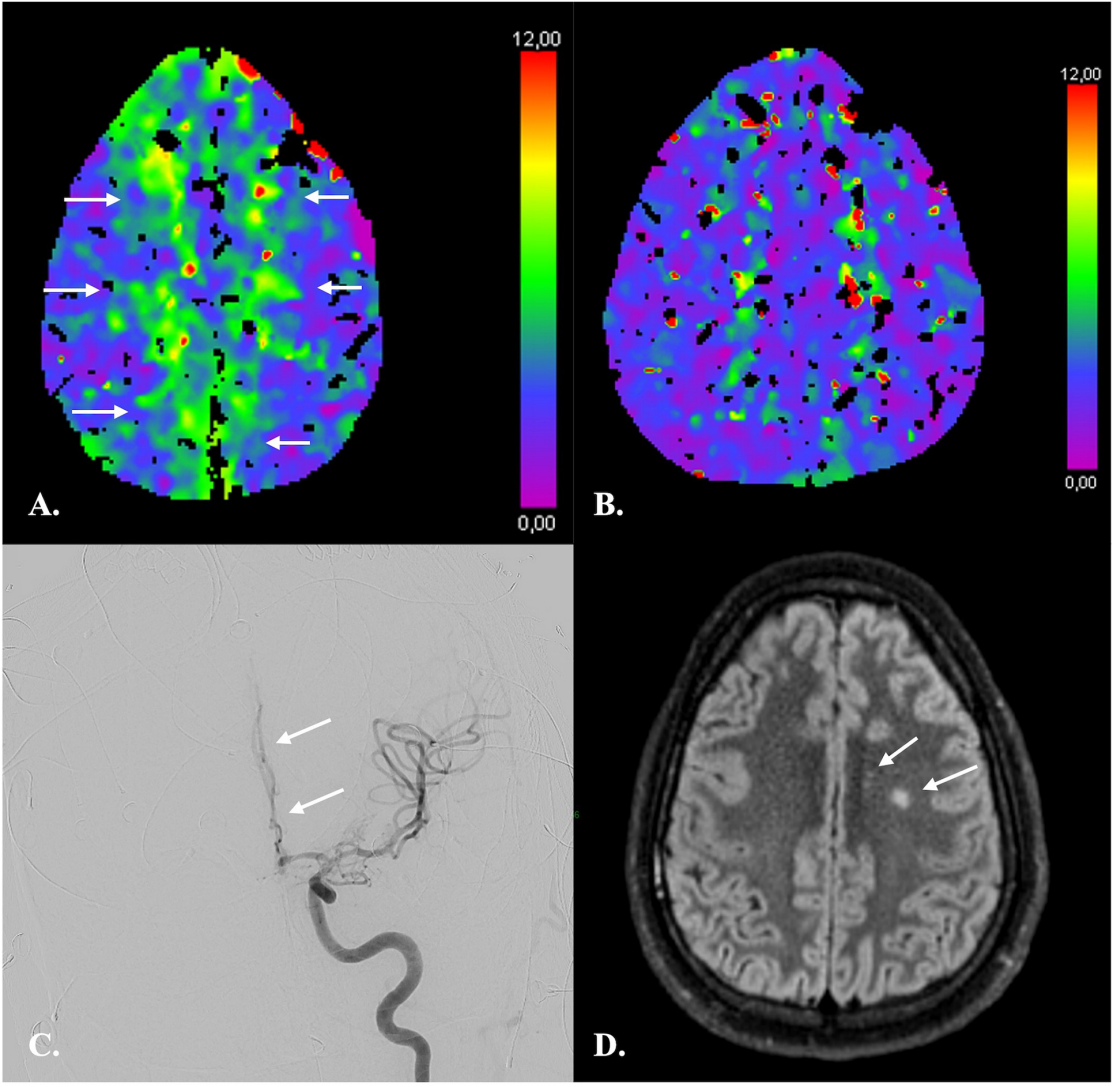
CTP imaging in the response assessment to ERT in a 26-year-old male with fisher 4 aSAH. (A) At day 11 after aSAH, CTP revealed a moderately increased TMax consistent with hypoperfusion of both ACA territories (*arrows*). (B) CTP following ERT on day 12 demonstrated almost complete regression of hypoperfusion. No new areas of hypoperfusion appeared. (C) DSA confirmed vasospasm of multiple intracranial arteries and in particular of the A2 segment of the left anterior cerebral artery (*arrow*). ERT consisted in intra-arterial injections of nimodipine and selective angioplasty of supraclinoid internal carotid arteries and M1 segment of the left middle cerebral artery as in Guenego et al. (96). (D) On MRI one month after aSAH, only two small infarcts of the left centrum semi-ovale were demonstrated.
